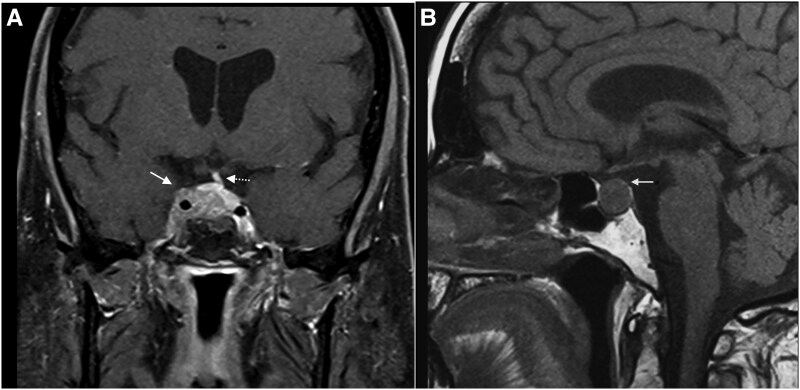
A, T1-weighted (T1W) postcontrast coronal magnetic resonance imaging (MRI) demonstrates a heterogeneous mass located on the right (solid arrow), infiltrating the cavernous sinus and encasing the right internal carotid artery (Knosp grade 4). The mass enhances to a lesser degree than the normal pituitary gland and the pituitary stalk is displaced to the left (dashed arrow). B, T1W postcontrast sagittal MRI demonstrates the expanded sella turcica and the mass, which extends into the suprasellar cistern (solid arrow), but does not compress or displace the optic chiasm.
